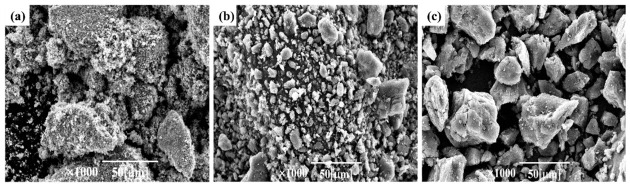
SEM images of the raw material (a), ultrasonic-assisted leaching residue (b), and conventional leaching residue (c).
